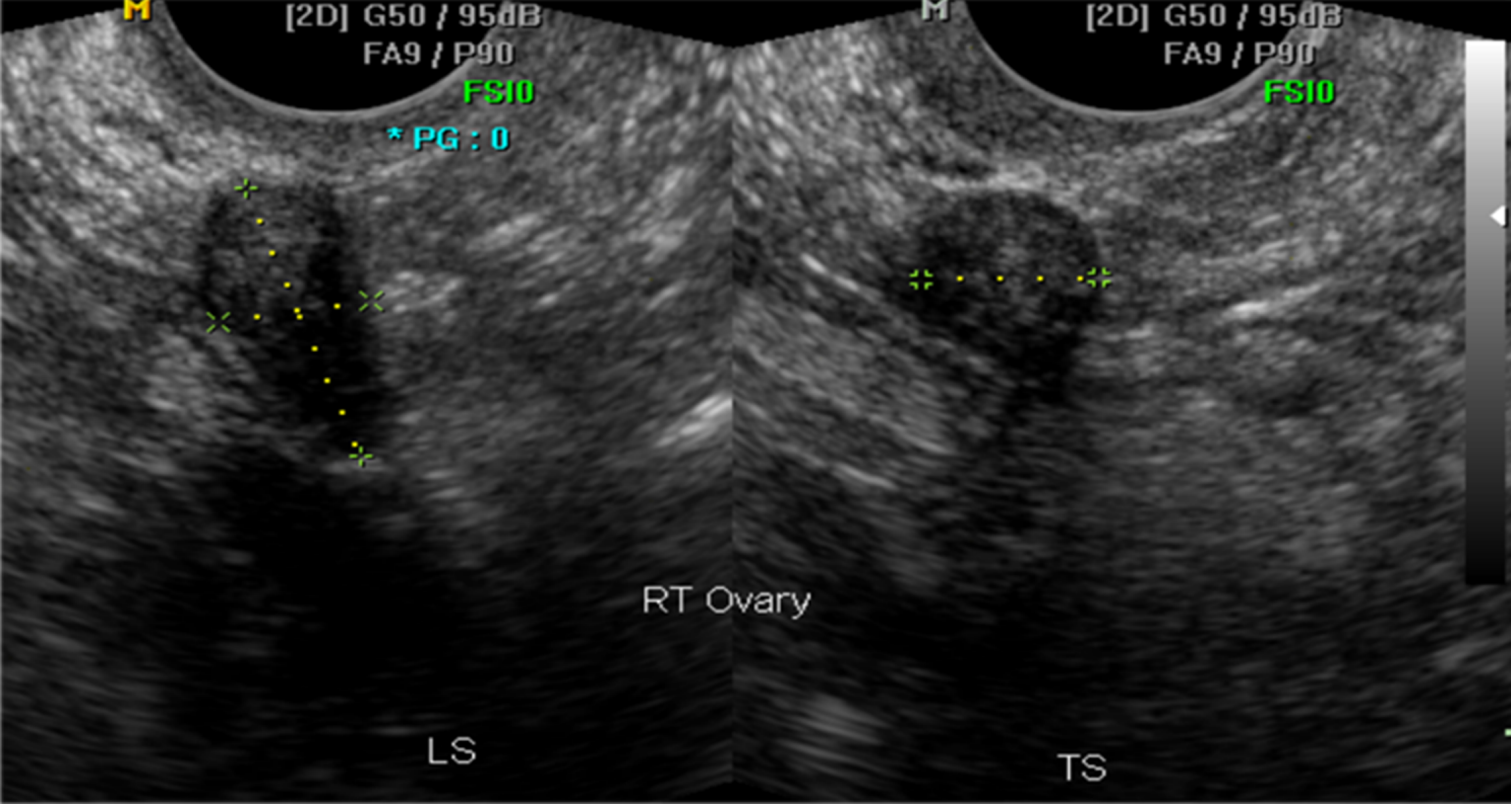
Longitudinal (LS) and transverse (TS) transvaginal ultrasound images of right ovary acquired by sonographer. This ovary was confirmed by the expert reviewer as normal and measured correctly.
